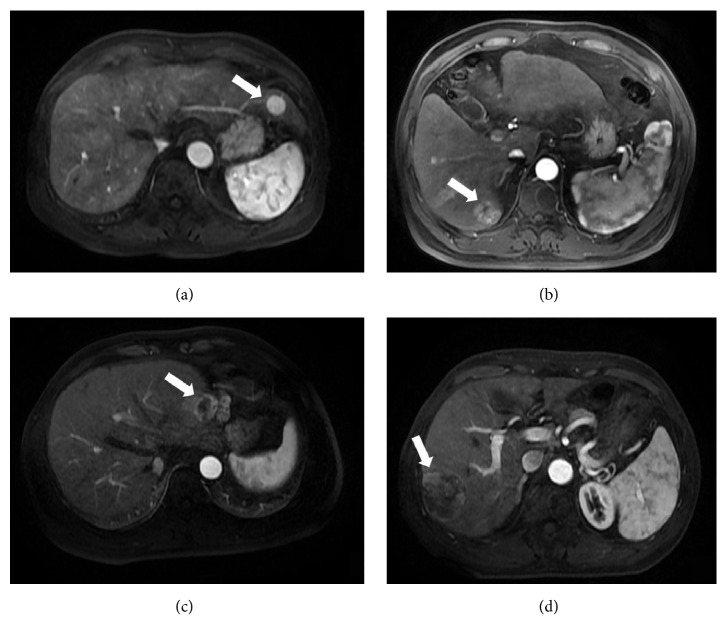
Axial late arterial phase DCE-MR images of four pathologically confirmed PD HCCs. The imaging appearances in the arterial phase of different PD HCCs varied, presenting with homogeneous hyperenhancement (a), heterogeneous hyperenhancement with a small area of necrosis (b), heterogeneous hyperenhancement with a large area of necrosis (c), and hyperenhancement in some parts of tumor (d).
